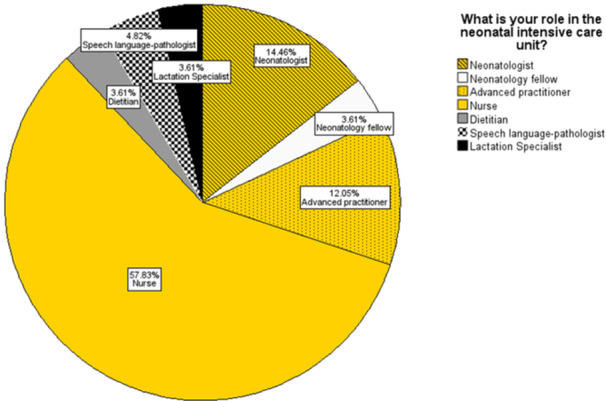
## 570 MANAGEMENT OF SMALL INTESTINAL BACTERIAL OVERGROWTH (SIBO) IN PEDIATRIC SHORT BOWEL SYNDROME (SBS) ‐ PERSPECTIVES OF AN INTERNATIONAL DELPHI PANEL
